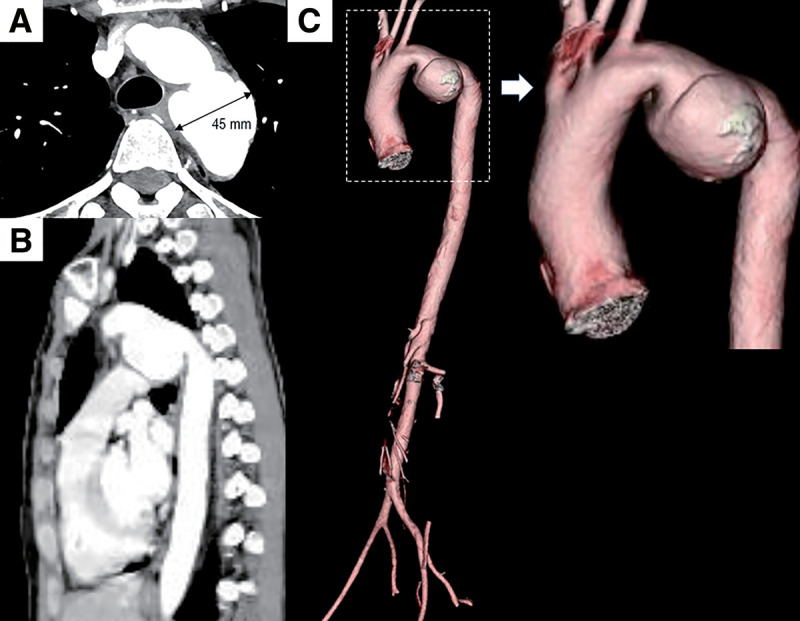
Preoperative computed tomography images. Axial (A), sagittal (B), and 3-dimensional reconstruction (C) views reveal a saccular aneurysm in the proximal descending thoracic aorta, without evidence of tortuosity in the thoracic or abdominal aorta.
